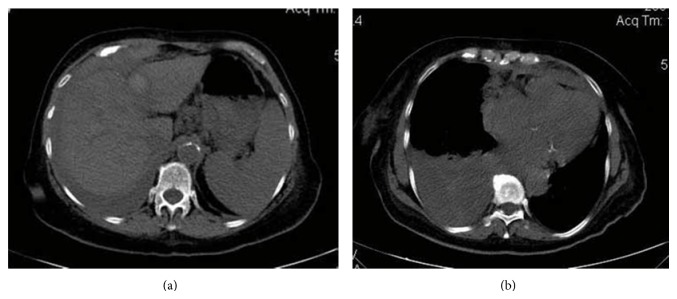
A chest CT was performed immediately which revealed a right pleural effusion with a distinct fluid level.
